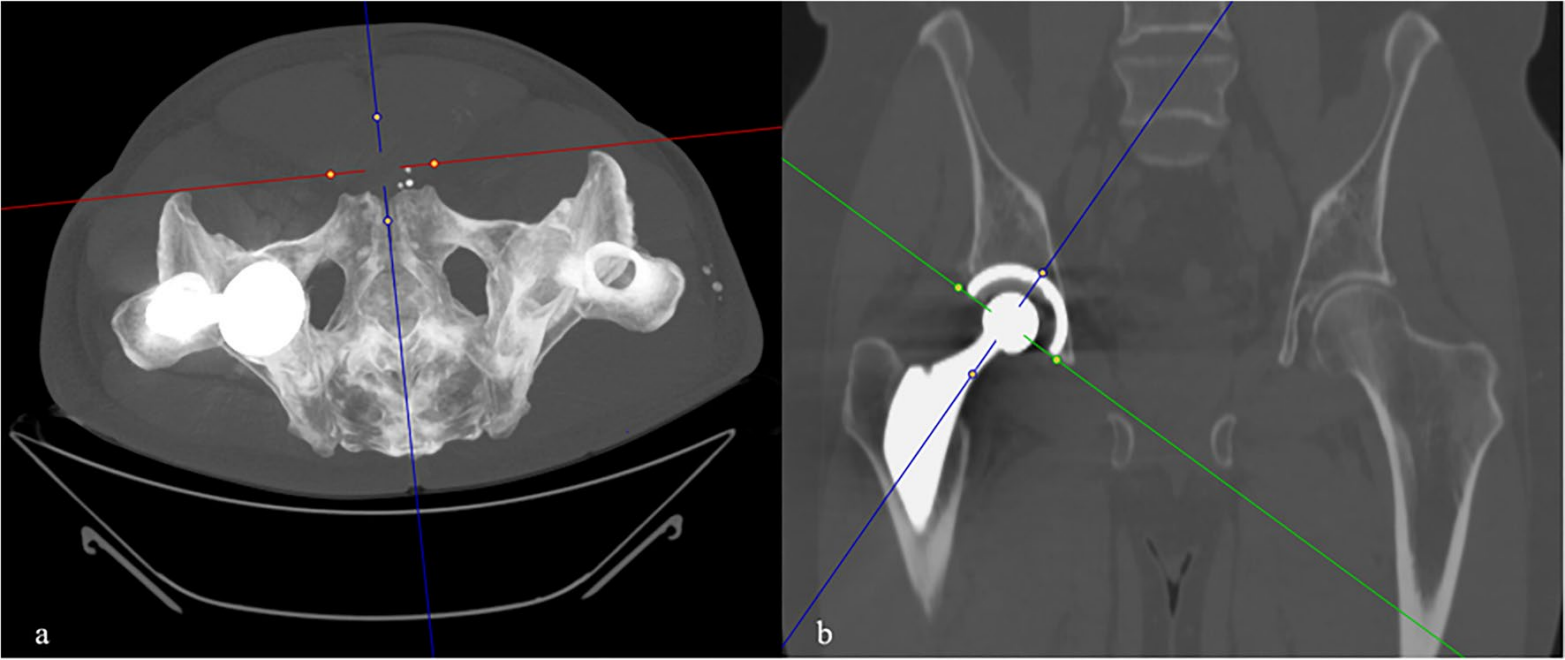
Multiplanar reformation of CT scans for corrected overhang distance measurement. Maximum intensity projection in the axial plane along the anterior superior iliac spine to correct for pelvic rotation (a) and angulation in the coronal plane along the plane of the cup face (b). The corrected overhang distance (OD_c_) is then measured on the corrected sagittal images parallel to the blue MPR reference line, as shown in Fig. 3. MPR, multiplanar reformation
